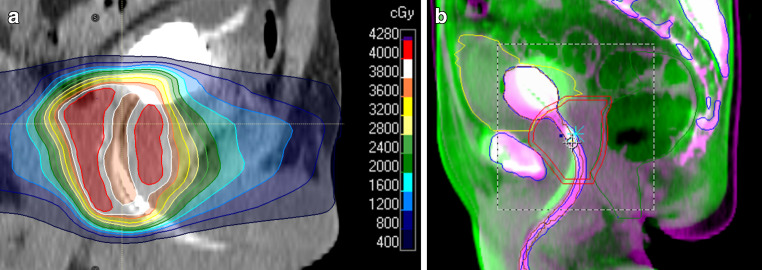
a Dose distribution as planned and overlay of cone-beam CT (green) and b planning CT (magenta) of an exemplary patient, registered to the transmitter
